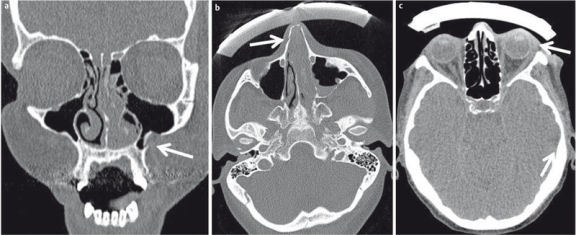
Low-dose CT of the midface using a 64-row dual-source CT scanner. a) 18-year-old female patient who fell on her face. Coronal reconstructed image with 3 mm slice thickness. The fracture (arrow) can be assessed precisely. b) 23-year-old patient with direct trauma to the head. Despite the lens protection device the fracture of the nasal bone is detected. c) 47-year-old patient with facial swelling on the left side. The preseptal cellulitis (arrow) is detected despite the lens protection device.
